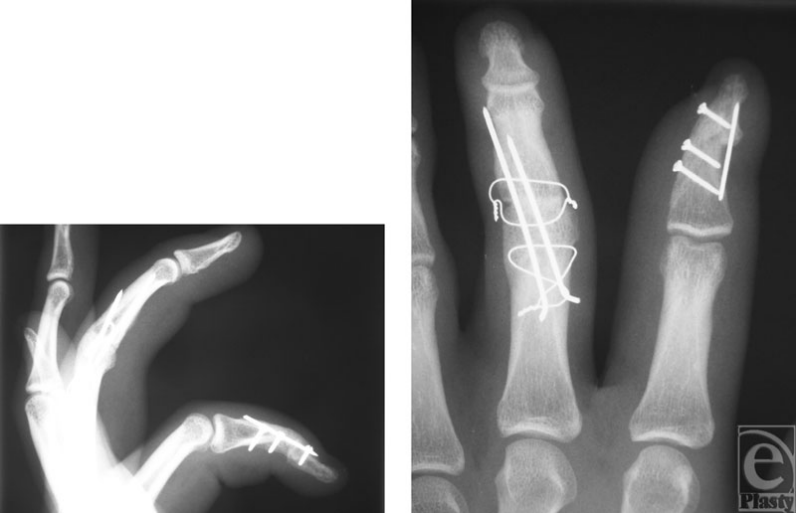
Postoperative radiograph at 6 months. Solidly consolidated arthrodesed joints after the interposition of iliac crest bone in the proximal interphalangeal joint of the middle digit and the distal interphalangeal joint of the index finger.
